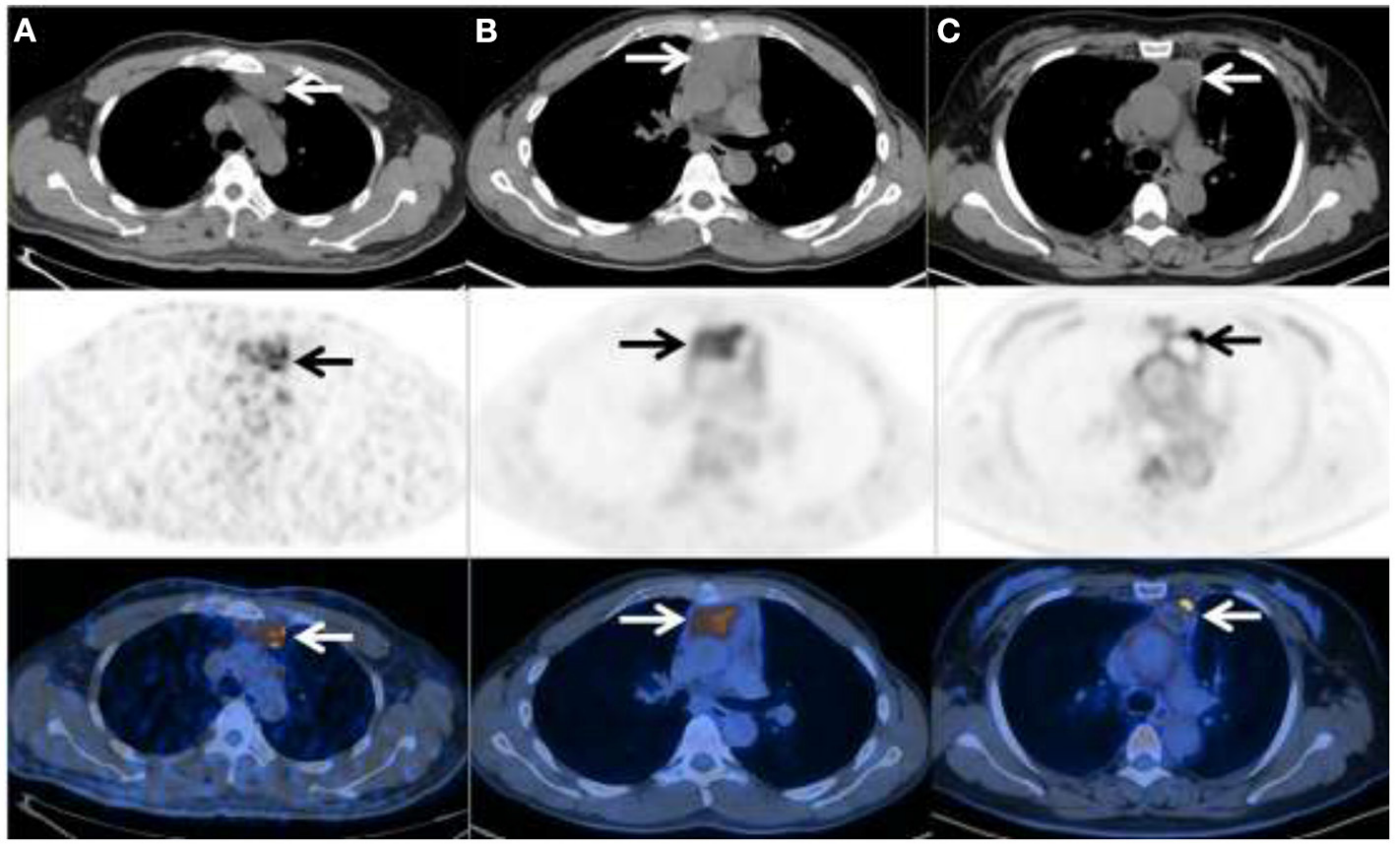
^18^F-FDG PET/CT in three patients with primary thymic MALT lymphoma. (A) Male, 47y, Ki-67 = 8%, SUV_max_ = 7.17, TLG = 99.85, MTV = 24.14 (B) Male, 31y, Ki-67 = 10%, SUV_max_ =6.08, TLG = 280.93, MTV = 80.02 (C) Female, 47y, Ki-67 = 8%, SUV_max_ = 12.34, TLG = 35.54, MTV = 5.14.
